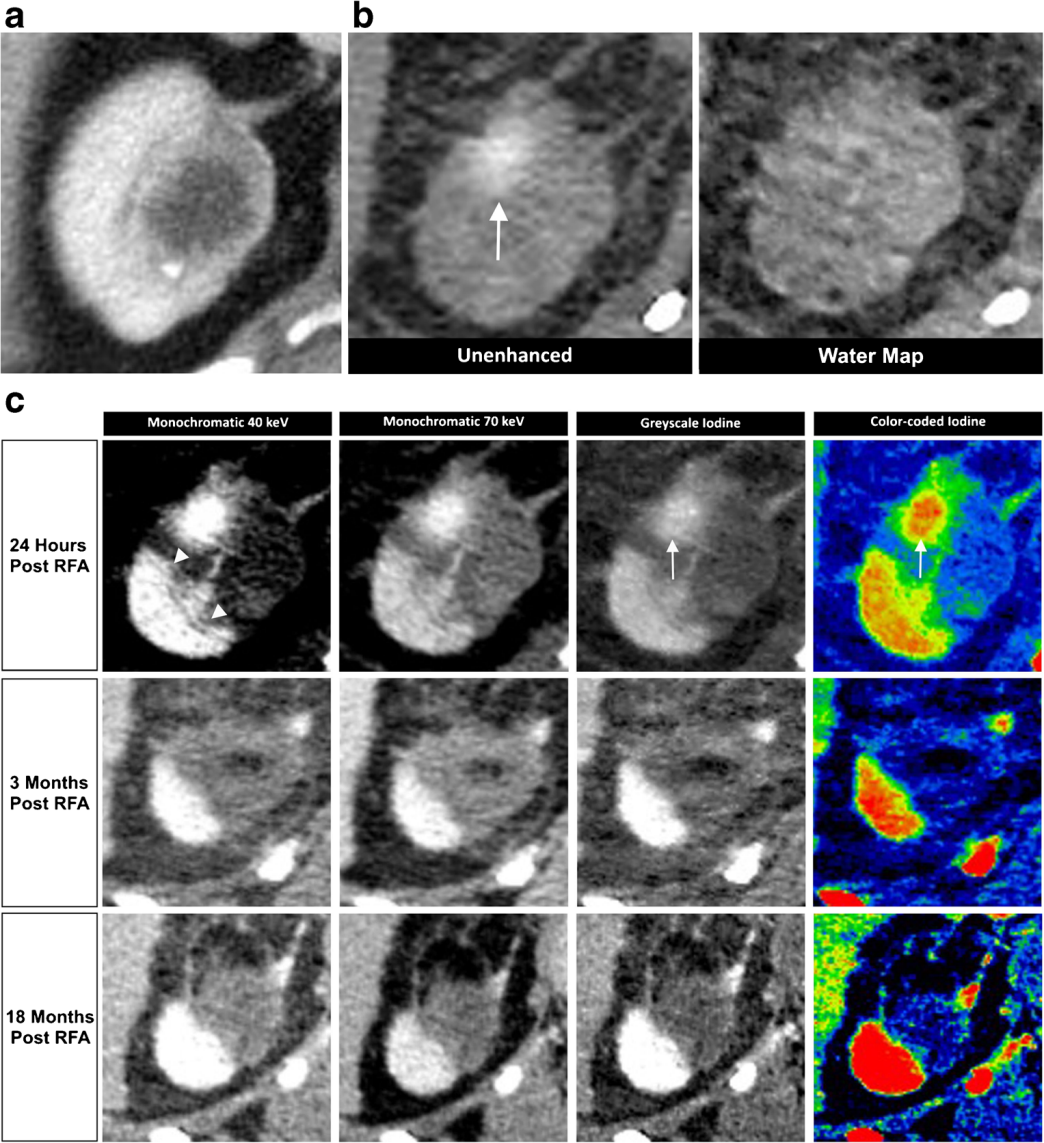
Renal cell carcinoma. A 40-year-old female is diagnosed with a suspicious lesion in the right kidney on ultrasound. After consulting her urologist, the patient opts for RF ablation. a CECT shows a thick-walled cystic lesion in the right upper pole, graded as a Bosniak 4 cyst. b The true unenhanced polychromatic CT image (left), 24 h post ablation, shows a hyperdense change in the ablation zone (*arrow*). This finding cannot be confirmed on the water map reconstructions. c The imaging appearance of the ablation zone 24 h (*first row*), 3 months (*second row*), and 18 months (*third row*) after RF ablation. On the 24-h post-ablation series, the delineation of the ablation zone is clearly observable on all reconstructed images; however, the 40 keV image is of superior quality when compared to the 70-keV image (*arrowheads*). On the iodine-coded images, the internal high attenuation is unexpectedly (no iodine content) still visible within the avascular zone (*arrows*). No suspicious nodular enhancement is observed at the border of the ablation zone. DECT from 3- and 18-month follow-up depicts an involution of the avascular zone. There is a loss of the internal high-attenuation changes. There is no evidence of local tumour progression
